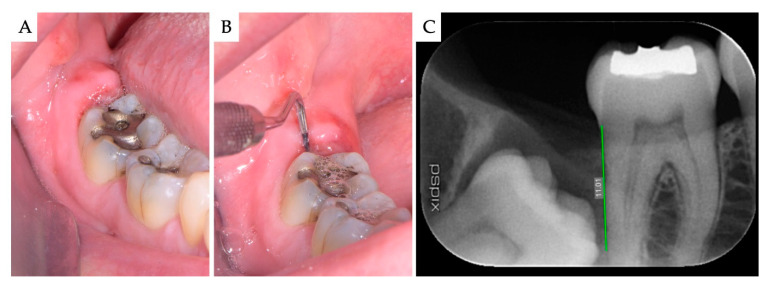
Case no 1. Impacted mesioangular M3 with pericoronitis. (A) Clinical view at presentation, showing the inflamed soft tissues distally to the M2; (B) PD was clinically recorded; and (C) the amount of BD was calculated on the digital periapical X-ray, using the measurement tool of the imaging software.
